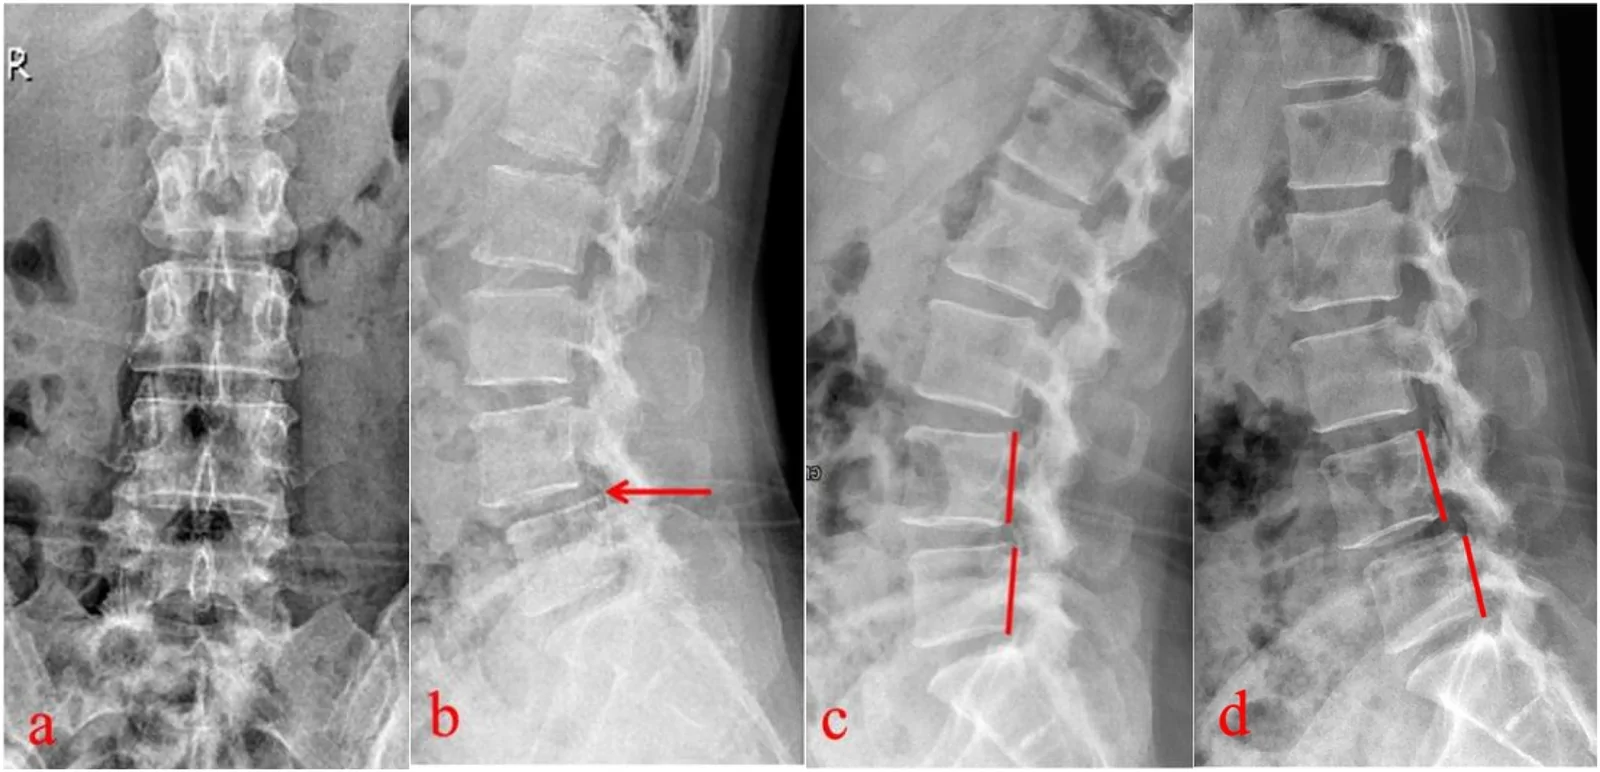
Preoperative lumbar radiographs demonstrated grade Ⅰ spondylolisthesis at the level of L4/5 on the lateral view (b) without scoliosis on the anteroposterior (AP) view (a) the lateral dynamic views revealed segmental instability at the L4/5 level (c,d).
